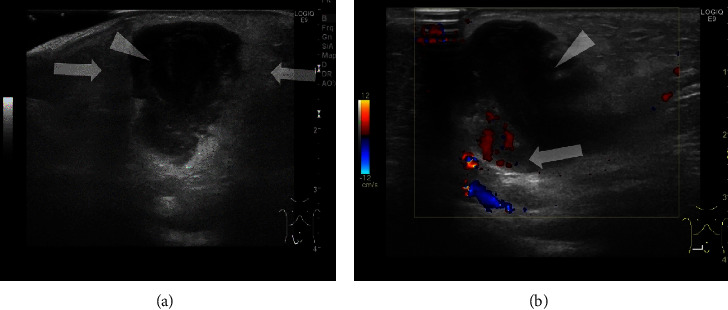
Case 4: a 2-month-old male with purulent lymphadenitis. (a) Sonogram of the right inguinal bulge shows a low-echoic lymph node (arrowhead) with surrounding effusion and high-echoic surrounding subcutaneous tissue (arrows). Therefore, purulent lymphadenitis was diagnosed. (b) Color Doppler sonogram at the inguinal lymph node shows the absence of vascular flow within the lesion (arrowhead). An adjacent enlarged lymph node is visualized (arrow).
